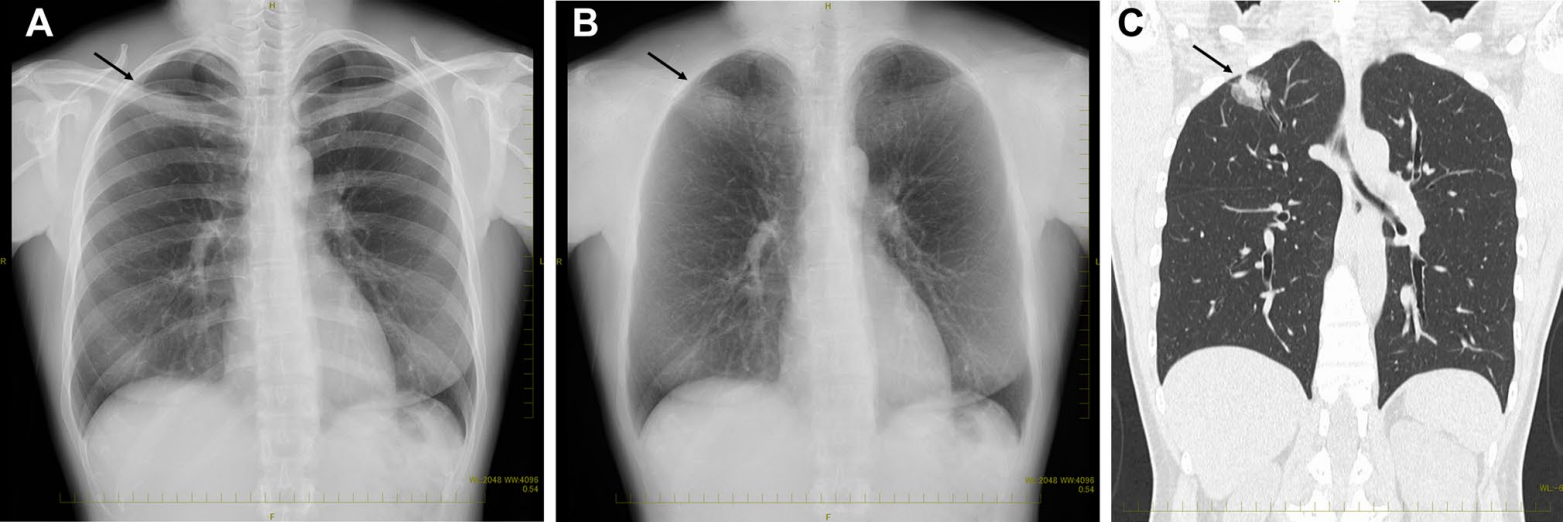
Nodule detection on chest radiograph with bone suppression by a commercially available AI model. A 42-year-old woman. A low radiolucent area is suspected on the right supraclavicular bone (arrow, A). However, it is difficult to distinguish between pulmonary and bone lesions. A pulmonary nodule hidden behind the right clavicle can be detected on the chest radiograph with bone suppression using a commercially available AI model (arrow, B). A part-solid ground-glass nodule with about 3 cm in diameter can be seen in the right upper lobe (arrow, C). This nodule was confirmed as a lung adenocarcinoma after surgery. *AI* artificial intelligence
